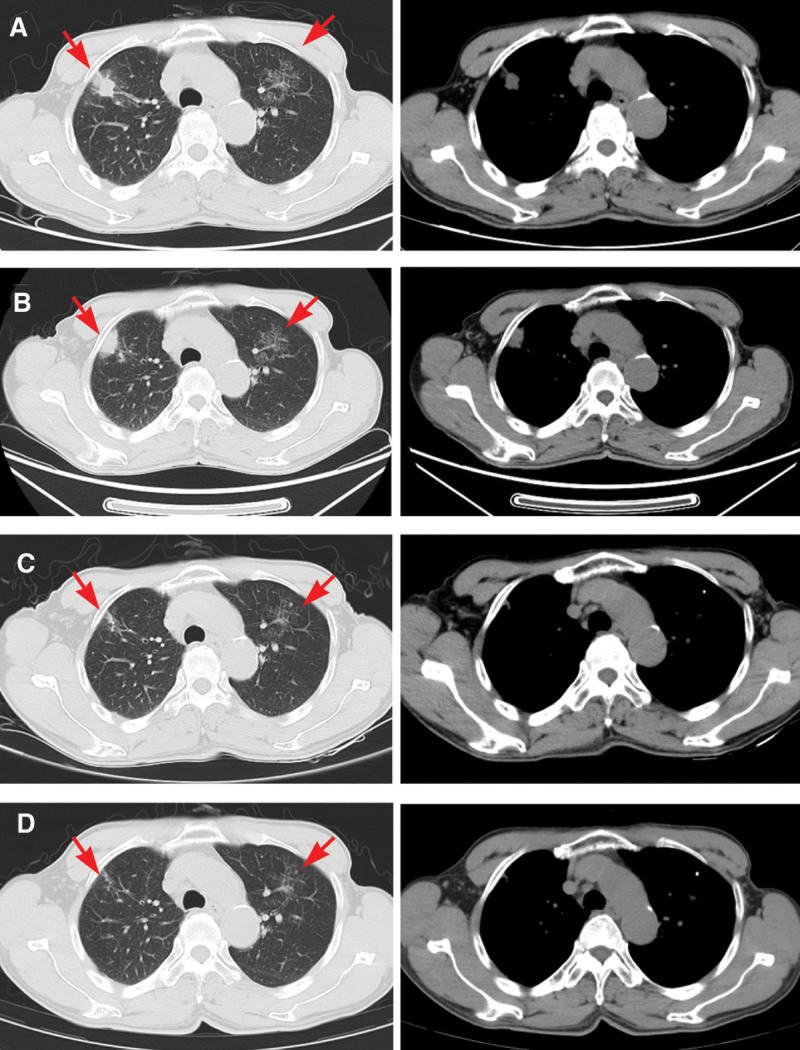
Computed tomography images of the patient. (A) Before 1 d in hospital, CT images showed irregular nodule in the left upper lobe and patchy opacity in the left upper lobe of the lung. (B) After 10 d in hospital, CT images at the initial diagnosis showed also irregular nodule in the left upper lobe and patchy opacity in the left upper lobe of the lung. (C) After received treatment for 6 wk, CT images showed a reduction in size of irregular nodule and decreased in extent of patchy opacity. (D) After received treatment for 9 mo, CT images showed a significant reduction in size of irregular nodule and with residual cord-like opacities remaining. CT = computed tomography.
